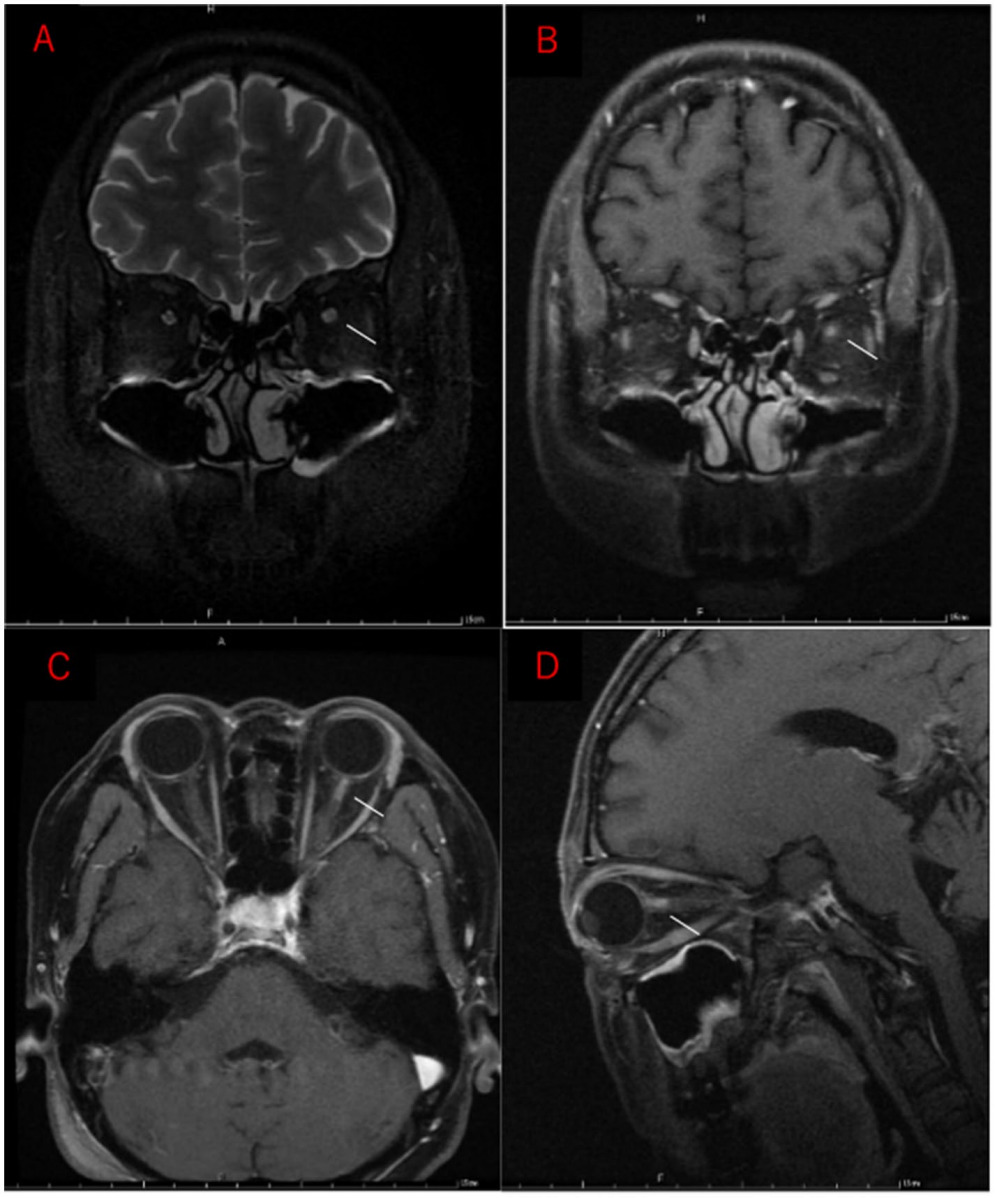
Orbital MRI performed 2 weeks after onset of left-eye vision loss. (A) Coronal T2-weighted image shows hyperintensity in the anterior intraorbital segment of the left optic nerve. (B–D) Post-contrast T1-weighted images demonstrate corresponding enhancement of the affected segment (white arrow).
